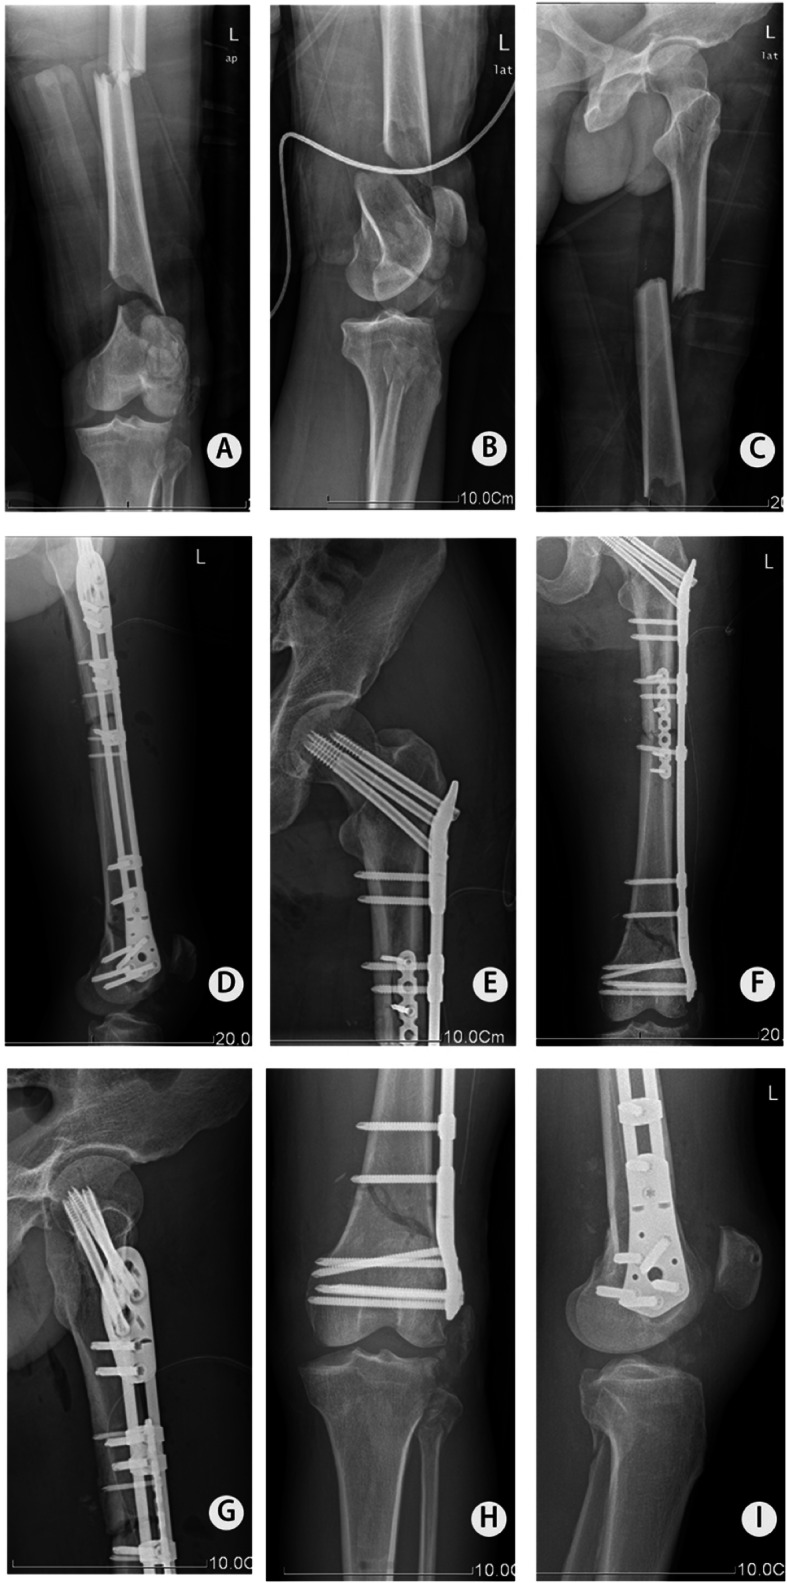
A 63-year-old male who suffered a motor vehicle accident and dignosed as ipsilateral femoral neck, shaft, and distal fracture. a–c X-ray at admission. d–i X-ray at 5 days after the operation
